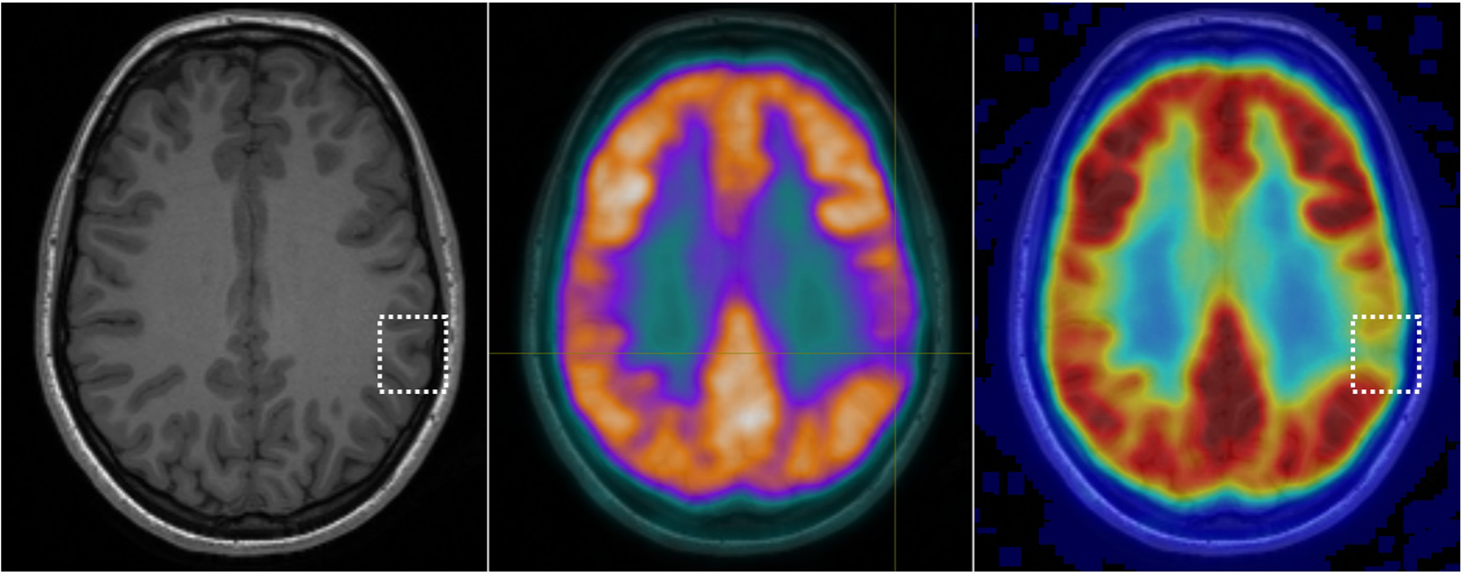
MRI and PET images for an individual with focal PET hypometabolism enabling detection of a subtle BOSD. MRI was initially negative in this patient, while a focal hypometabolism was detected on PET. Subsequent coregistration of MRI and PET enabled detection of a small BOSD in this patient. This patient was seizure free following focal resection of the subtle BOSD
